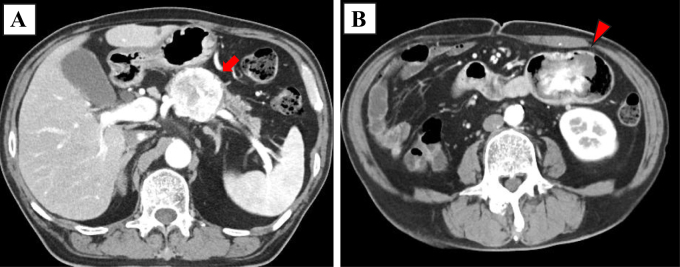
Contrast-enhanced computed tomography findings. (A) Contrast-enhanced computed tomography showing a hypervascular mass (arrow) in the pancreatic body measuring 50 mm in diameter. (B) Axial contrast-enhanced computed tomography image showing a 50-mm hypervascular mass (arrowhead) in the proximal jejunum near the ligament of Treitz.
